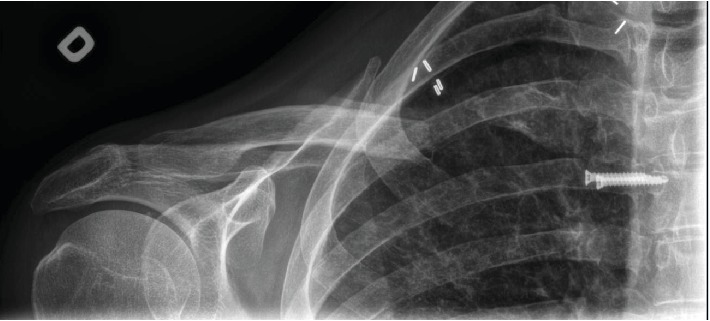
Clavicle radiograph three years postreconstruction surgery. Two remaining screws are superficial to the sternum.
